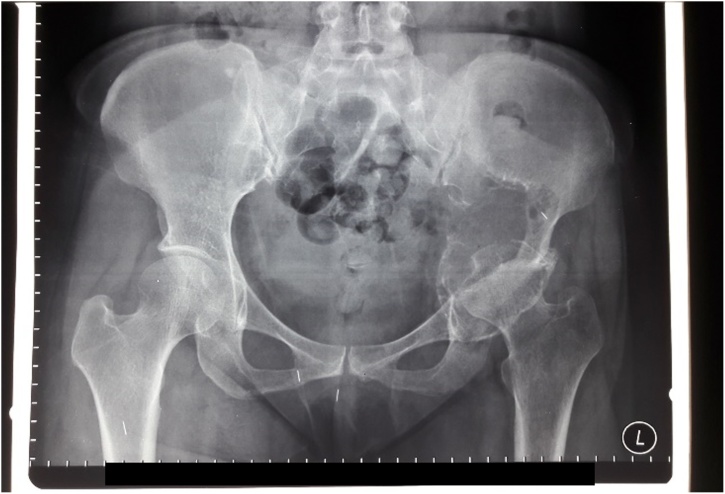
Pelvic X-Ray Examination Showing Lytic Lesion on the Left Ilium and Ischium.
